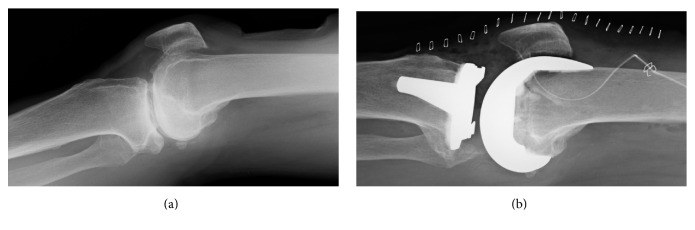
Lateral radiographs of the knee. (a) Preoperative passive knee extension without anesthesia. (b) Passive extension under postoperative anesthesia immediately postoperatively. Full knee extension was restricted preoperatively but it was possible immediately postoperatively.
